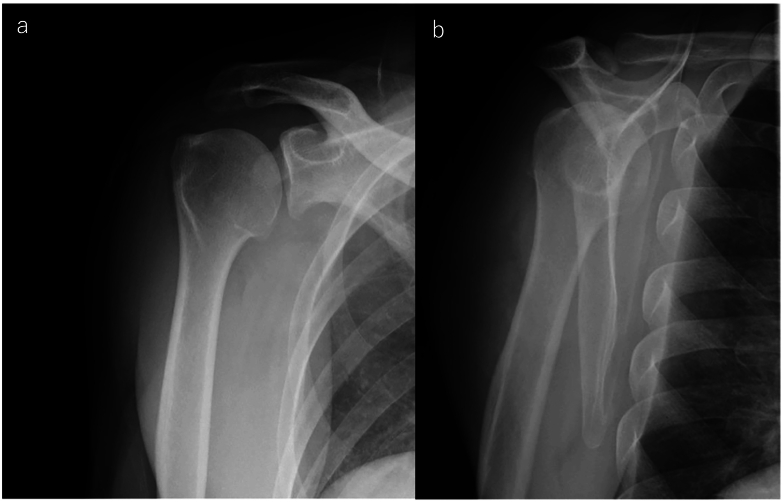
Postoperative shoulder radiographs at six months showed no evidence of a new loose body. a: Anteroposterior view. b: Scapular Y-view.
